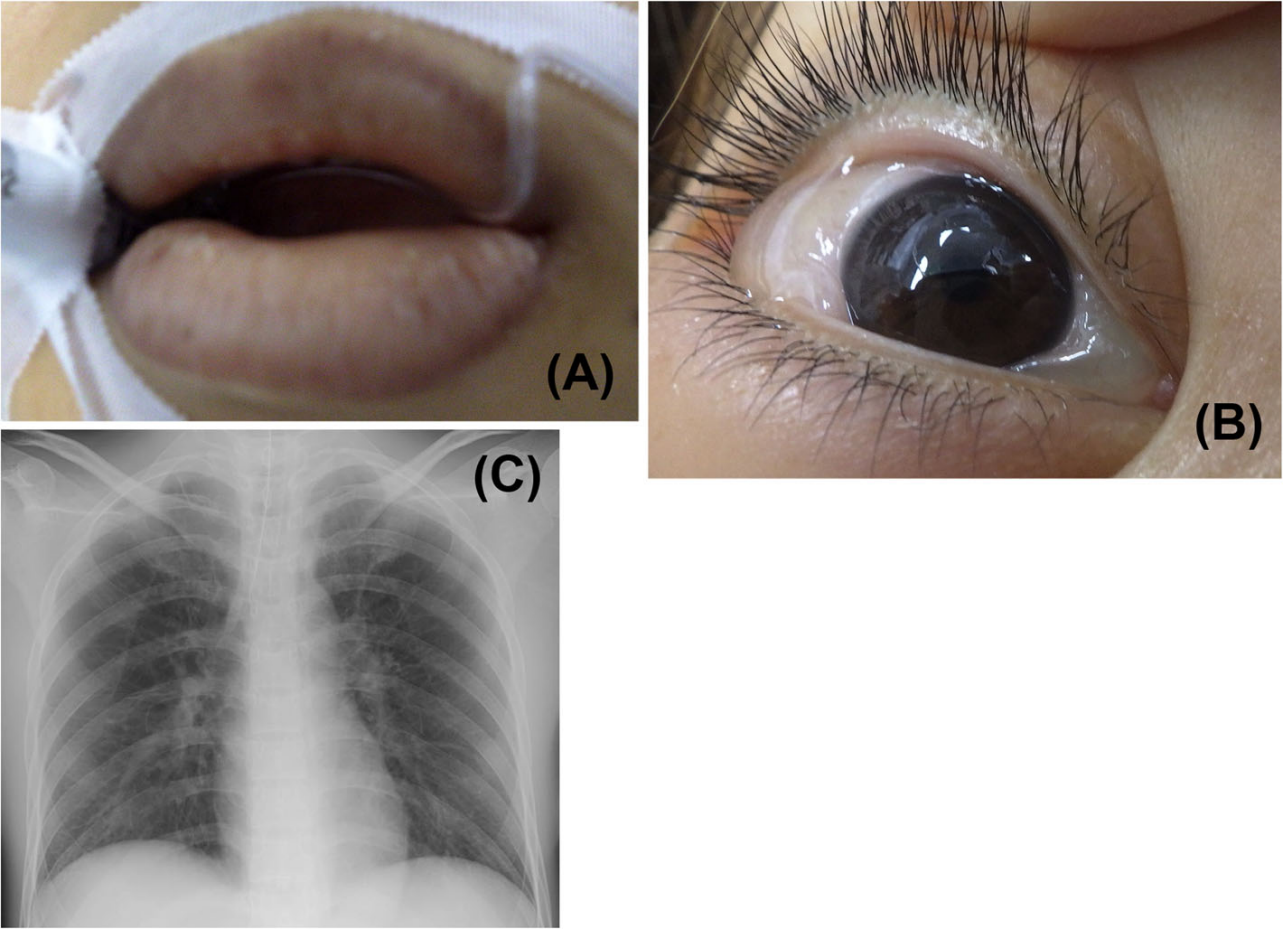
Edema of lips (a) and conjunctiva (b), and chest X-ray (c) at the end of the operation
